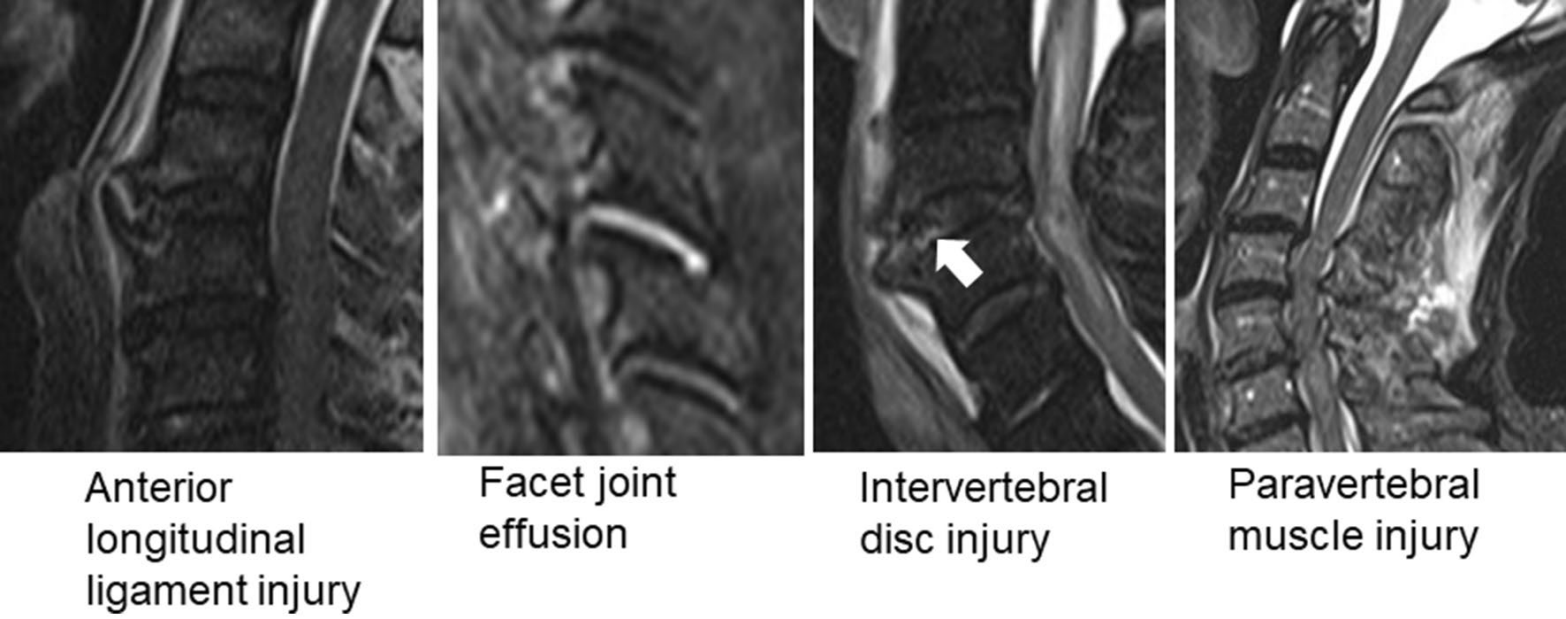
Assessment of cervical spine injury via magnetic resonance imaging (MRI, anterior longitudinal ligament injury, facet joint effusion, intervertebral disc injury (white arrow), and paravertebral muscle injury were assessed on T2-weighted MRI images of the cervical spine or short tau inversion recovery images.
